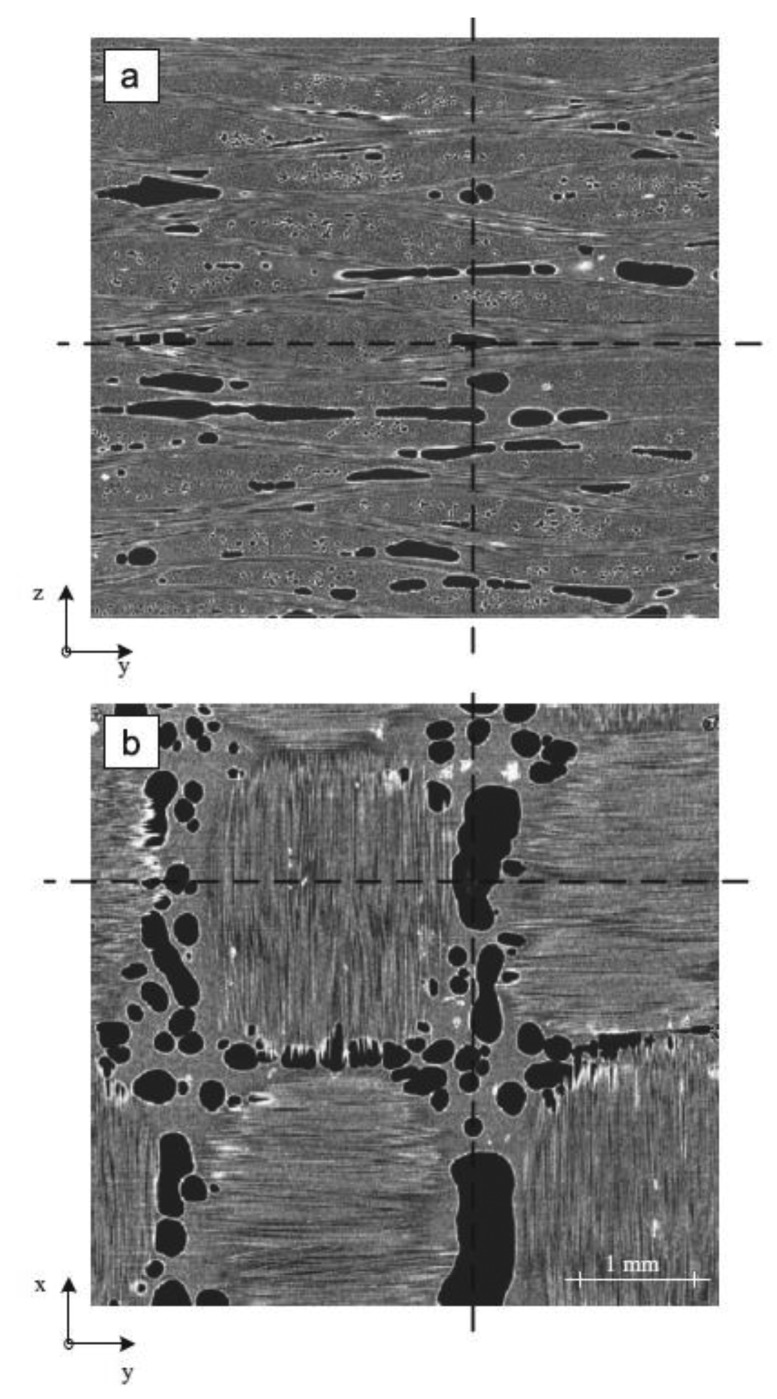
Computed tomography cross-section plot of a typical void structure in a woven CFRP (frame with permission from [140]).
